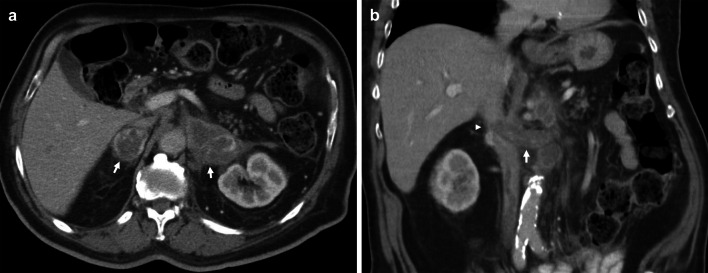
Adrenal metastases with suprarenal IVC tumour thrombus in a patient with small cell lung carcinoma primary. a Axial CT shows bilateral suprarenal lesions with heterogeneous peripheral enhancement in keeping with bilateral adrenal metastases. b Coronal CT demonstrates extension of tumour into the left renal vein (arrow) and suprarenal IVC (arrowhead). The heterogeneous appearance of the suprarenal IVC is due to a combination of tumour thrombus and flow artefact
